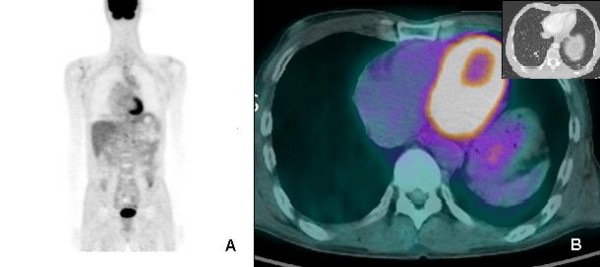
A: PET CT demonstrating no metastatic lesion after 8^th ^FOLFOX4 cycles. B: PET CT demonstrating no FDG upake in the lung include left lower lobe.
